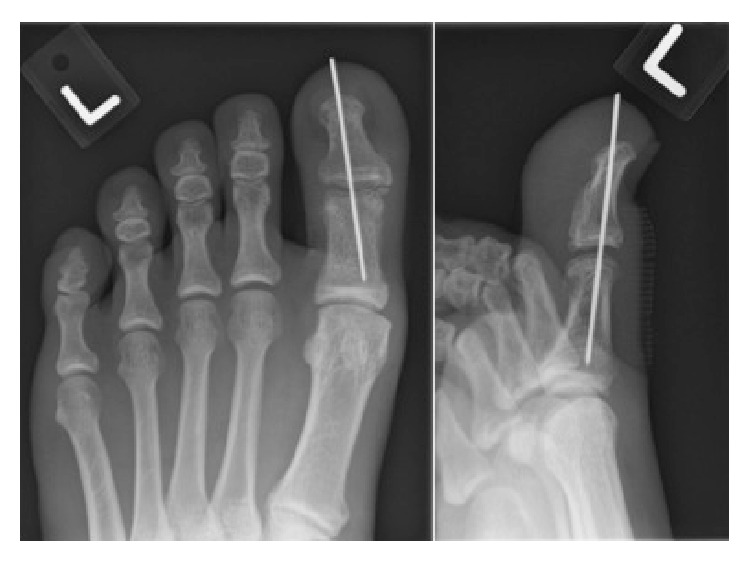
These are the radiographs obtained 2 weeks postoperatively showing an enlocated IP joint of the left great toe with the Kirschner-wire well placed.
